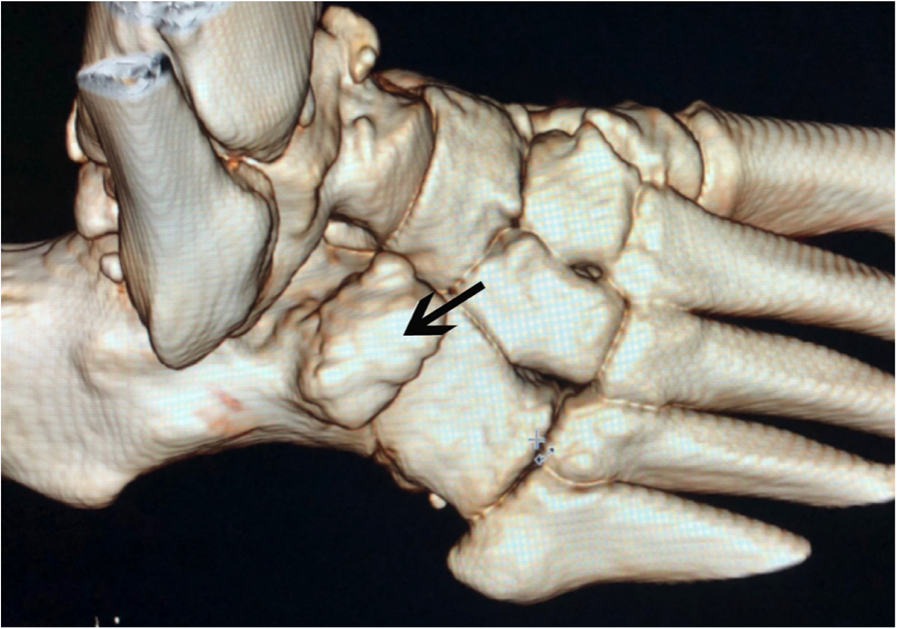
Three-dimensional volume-rendering CT image of the right foot. The arrow show the large calcaneus secondarius
